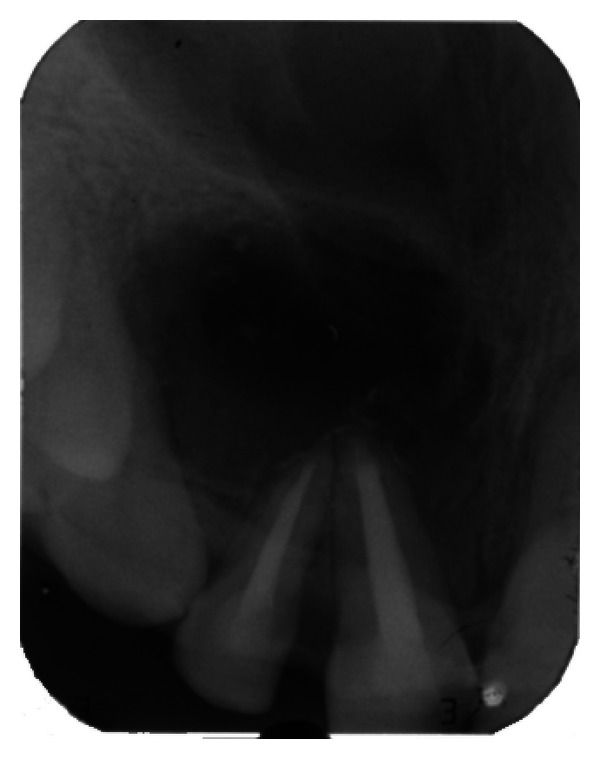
1-month followup IOPA radiograph.
